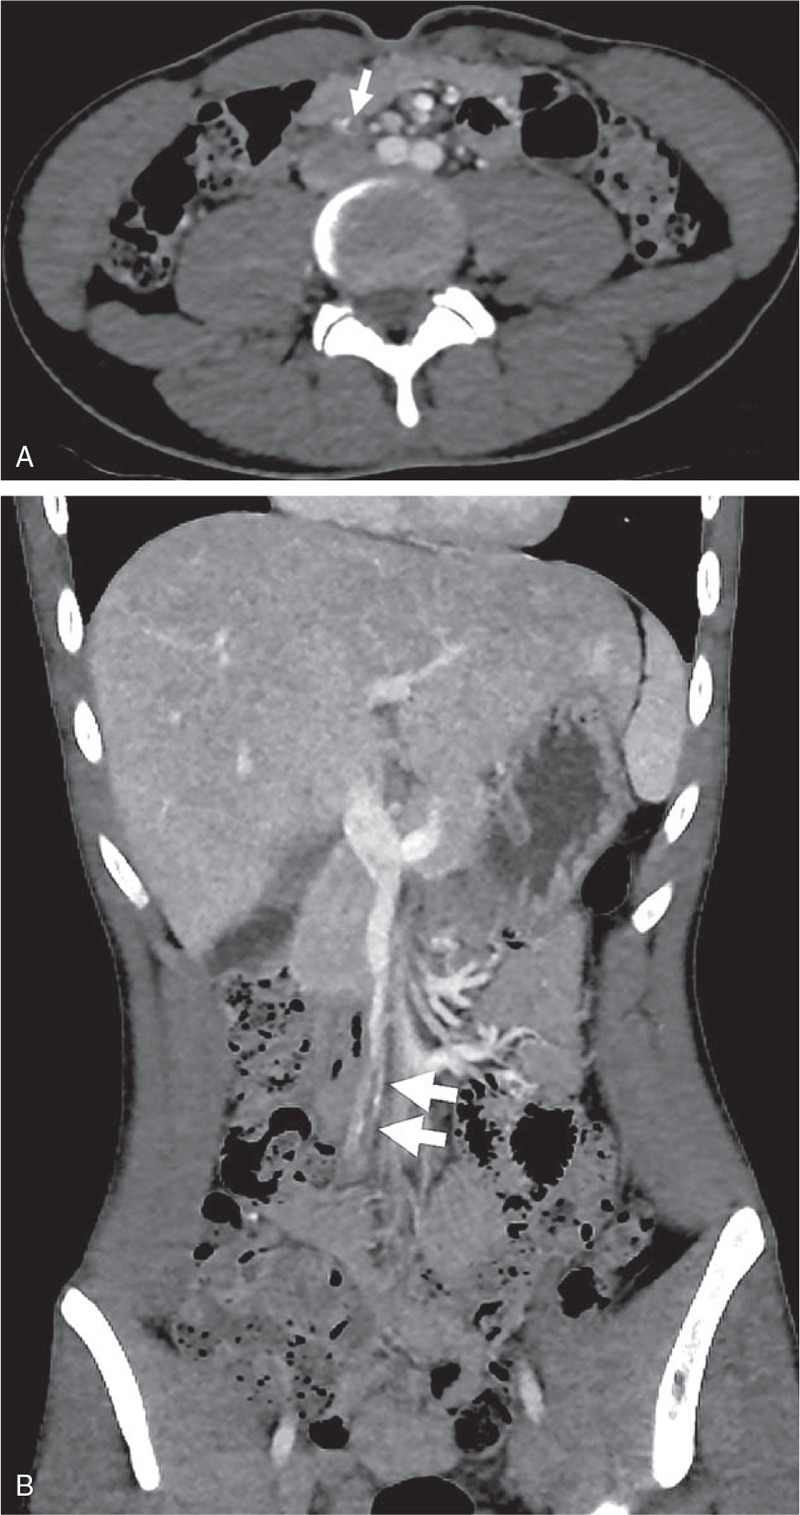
Computed tomography scan demonstrated a focal thrombotic occlusion of the superior mesenteric branch without associated bowel change (A)(B) (see arrow).
